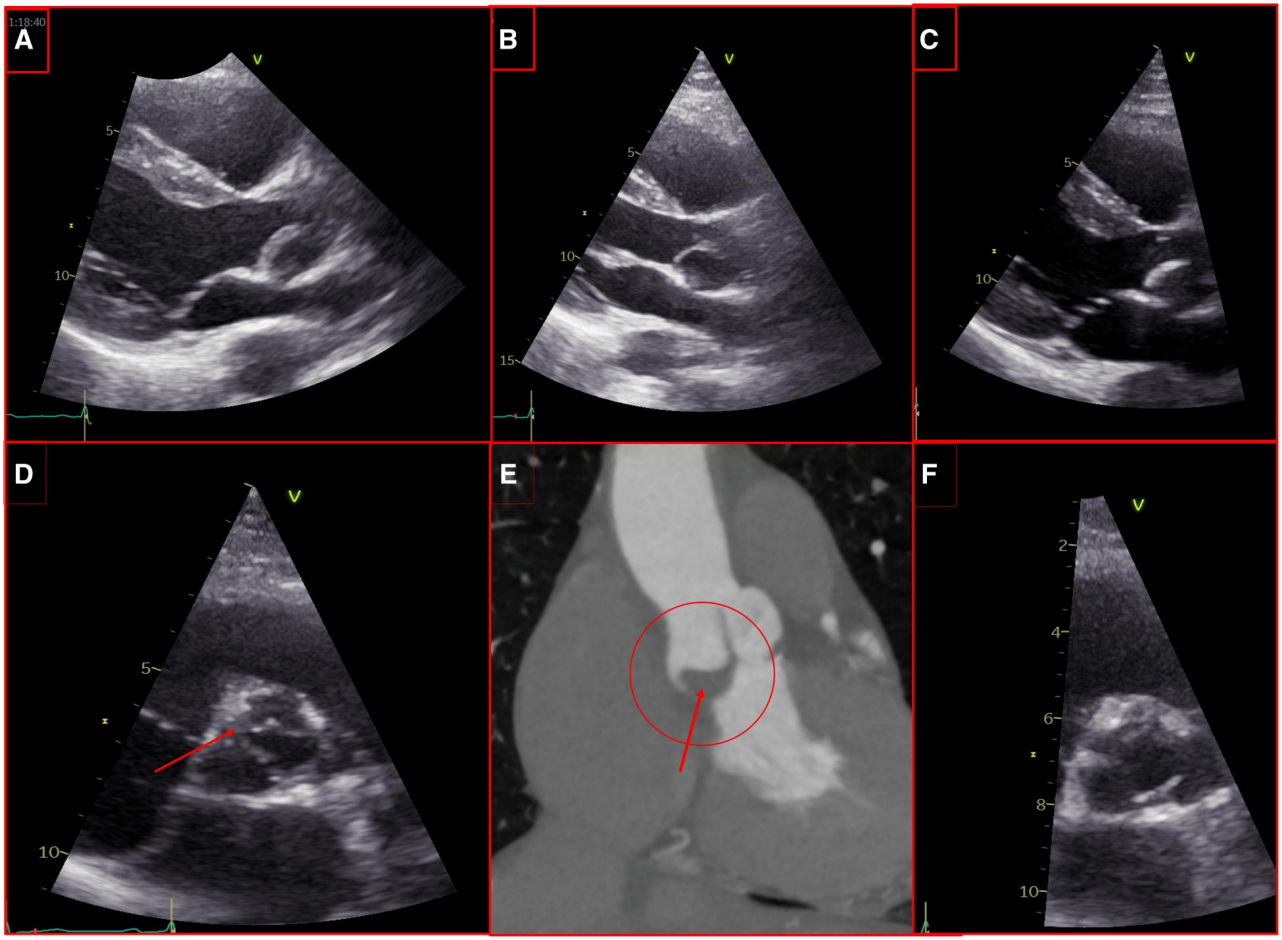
(A–C): First patient TTE: (A): at 12 months: leaflet thickening. (B): 4 months after anticoagulation: leaflet thickness restoration. (C): at 59 months: rethickening. Second patient TTE and Ct.: (D, E): TTE and CT at 24 months: thickening and thrombosis (red arrow). (F): TTE 4 months after anticoagulation: normal thickness.
